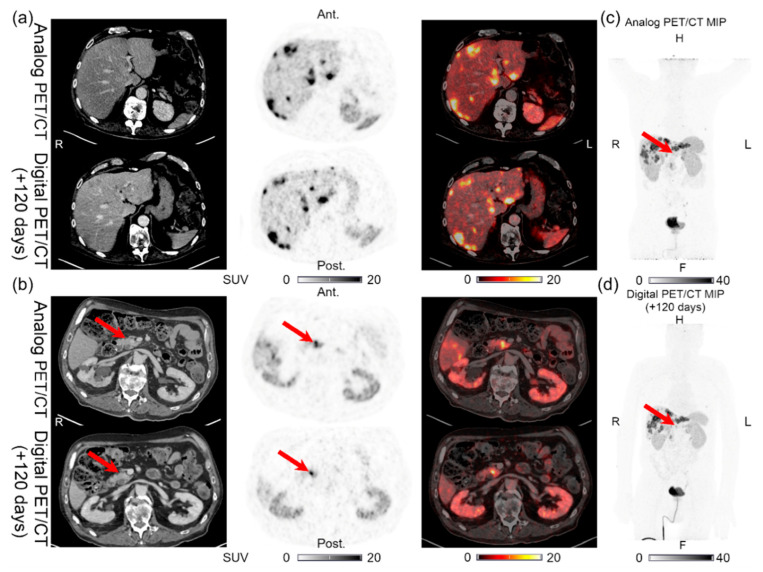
Patient 2: (a) multiple liver lesions and (b) single pancreatic lesion with structural CT correlates found on both the analog PET and the digital PET performed 120 days later. (c) MIP for the analog PET and (d) MIP for the digital PET, with arrows showing the pancreatic lesion location in (b).
